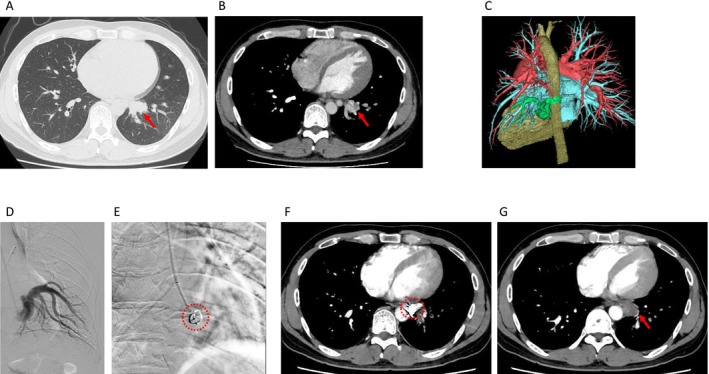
(A, B) Computed tomography (CT) images showing a nodular, mass‐like lesion in the left lower lobe, which corresponded to the anomalous systemic artery (red arrow). (C) Posterior oblique three‐dimensional CT angiographic reconstruction showing an anomalous systemic artery (green) arising from the descending thoracic aorta and supplying the left basal segment. (D, E) Angiographic findings during transcatheter embolisation: Selective angiography showing the anomalous systemic artery and its distal branches (D), and completion angiography confirming occlusion of the anomalous artery, with the embolisation device indicated by the red circle (E). (F, G) Follow‐up contrast‐enhanced CT obtained 1 week after the procedure. (F) Stable position of the embolisation device without migration (red circle). (G) No contrast enhancement of the anomalous artery (red arrow), consistent with thrombosis.
